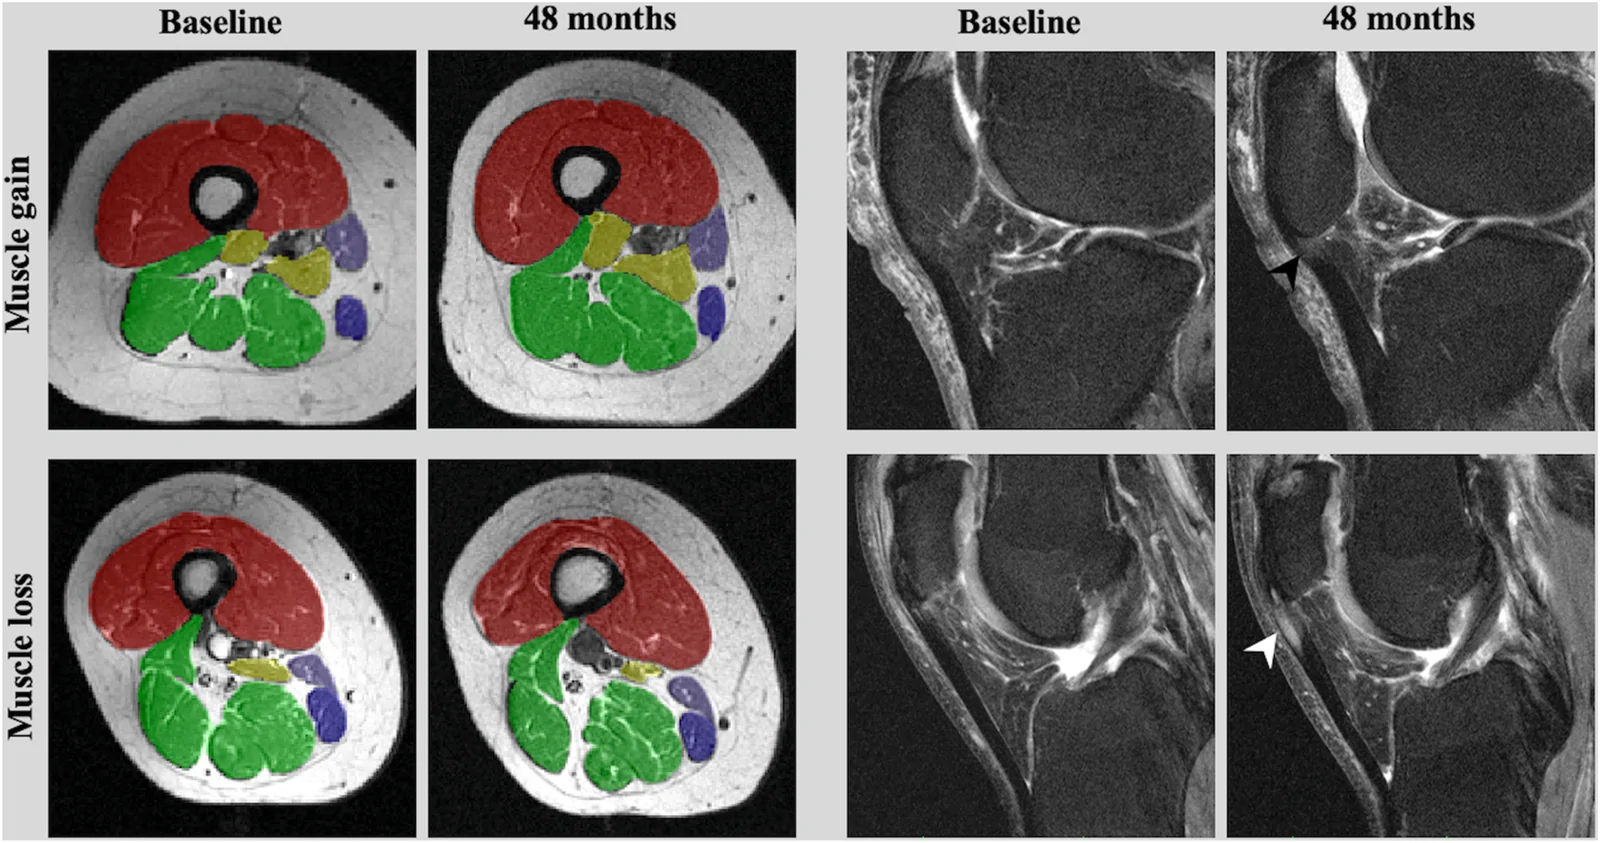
Imaging examples of changes in the patellar tendon. Left: Axial T1-weighted MR images of the right thigh with artificial intelligence-enabled muscle segmentation at baseline and after 48 months. Right: Sagittal intermediate-weighted fast spin-echo fat-suppression sequence of the right knee at baseline and after 48 months. The patient with muscle gain (predominantly of the quadriceps (red) and hamstrings (green), with an approximately 8% increase in total muscle volume over 48 months) was a 78-year-old man with a slight decrease in knee pain over 48 months (WOMAC pain subscore at baseline of 3 and after 48 months a score of 2) showed a new signal abnormalities around the patellar tendon at 48 months (black arrowhead), with a baseline WORMS patellar tendon subscore of 0 and 1 at 48 months). The patients with muscle loss (predominantly of the quadriceps (red), with an approximately 8% decrease in total muscle volume) was a 66-years-old man mild knee pain at baseline (WOMAC pain subscore of 1) and an increase of knee pain over 48 months (WOMAC pain subscore of 7 at 48 months) showed an increased tendinopathy of the patellar tendon (white arrowhead), with baseline WORMS patellar tendon subscore of 0 and 2 at 48 months.
